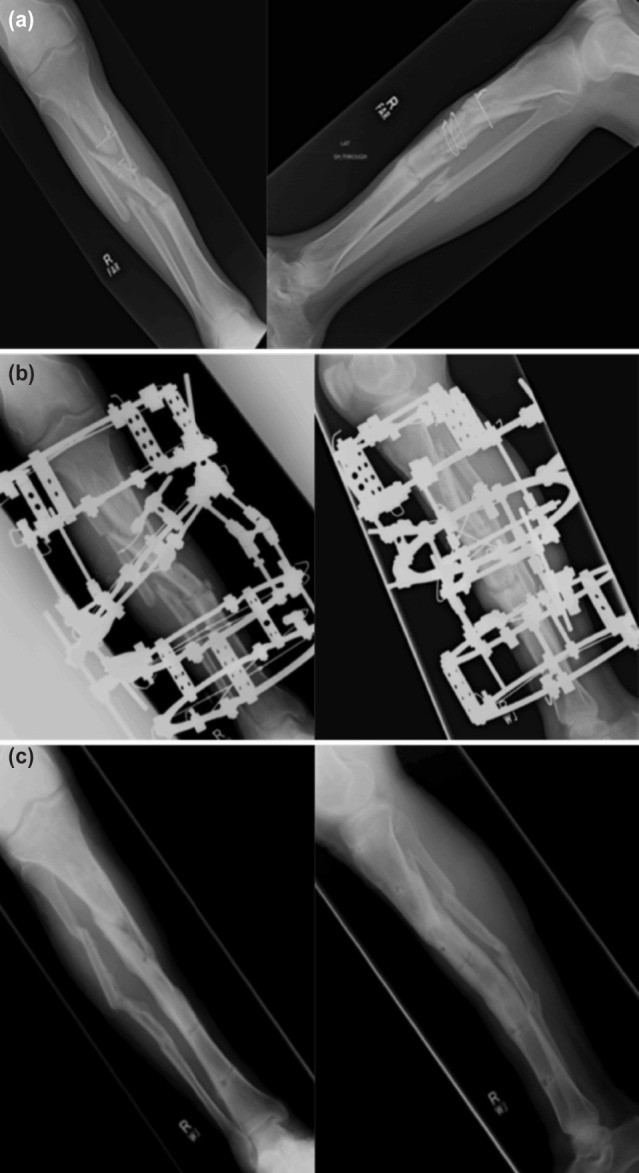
Radiographs of Patient 1. (a) Pre-operative antero-posterior and lateral views showing tibial non-union. (b) Post-operative antero-posterior and lateral views after Ilizarov application. (c) Antero-posterior and lateral views at the time of final follow-up showing bony union.
